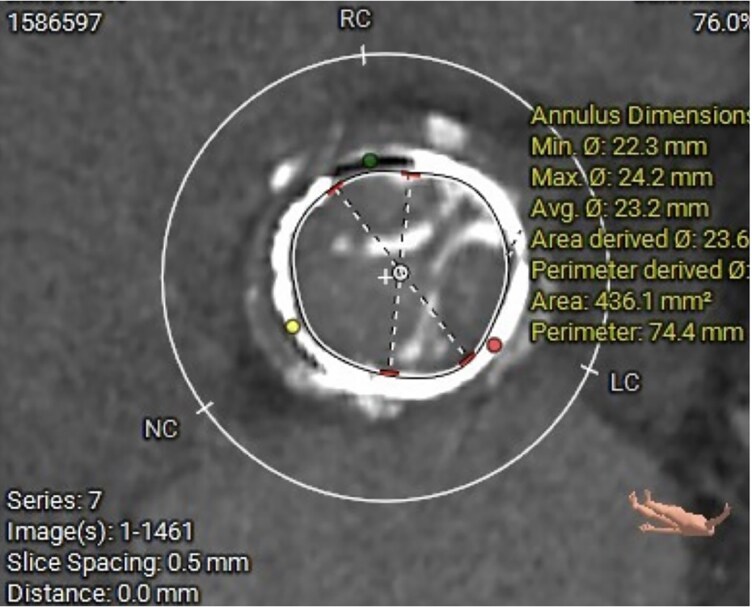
Pre-procedural CT assessment of the aortic root and bioprosthesis.
